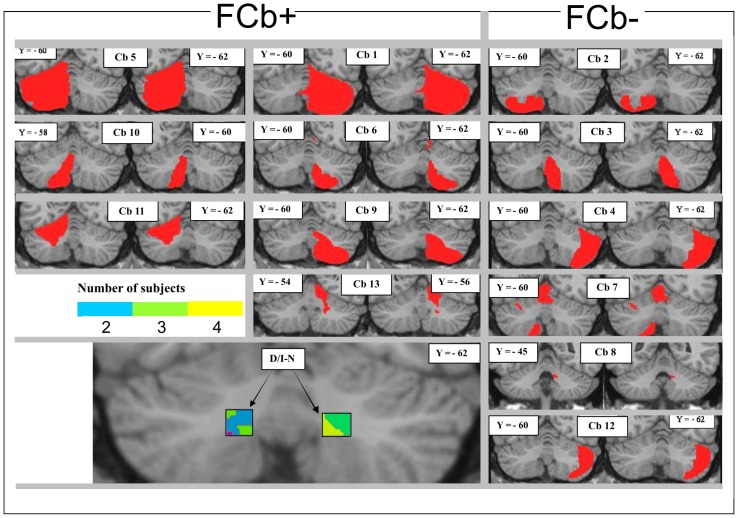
Lesion reconstruction in FCb+ and FCb− patients. Each individual lesion is presented as an overlay of 2 representative coronal MRI T1-weighted templates in stereotaxic space after spatial normalization. The bottom left of the figure shows lesion overlap between patients and a reconstruction of the 3-D probabilistic map (51% to 60%) of right and left dentate/interposed (D/I-N) cerebellar nuclei. The color code indicates the number of subjects whose lesions overlapped with D/I-N nuclei.
